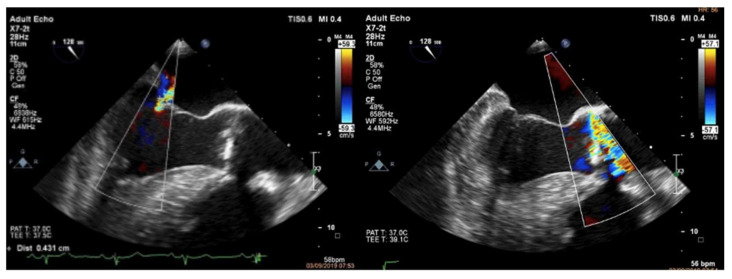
Preprocedural transesophageal echocardiography—moderate mitral regurgitation.
